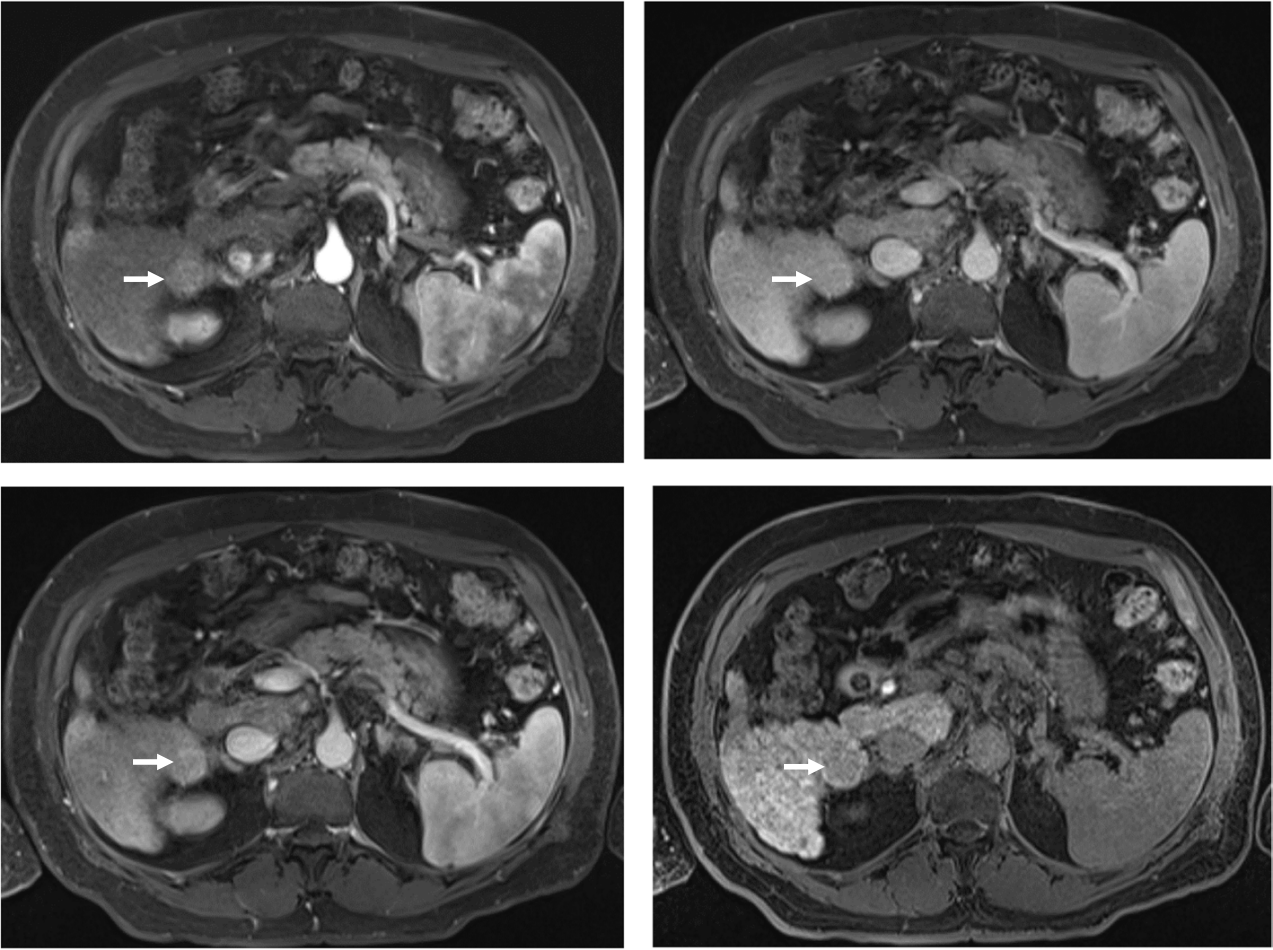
A 70-year-old male with chronic hepatitis B-related cirrhosis. Axial T1-weighted post-gadolinium fat-saturated images demonstrate a segment 6, 27 mm lesion with arterial-phase hyperenhancement (A), no washout on portal venous (B) or hepatic venous (C) phases, and hypointensity on hepatobiliary phase (D). The lesion would not count as having a washout as per LI-RADS (LI-RADS 4) and EASL (non-HCC) guidelines but would be considered to have washout as per APASL and KLCA guidelines (HCC as per both guidelines)
